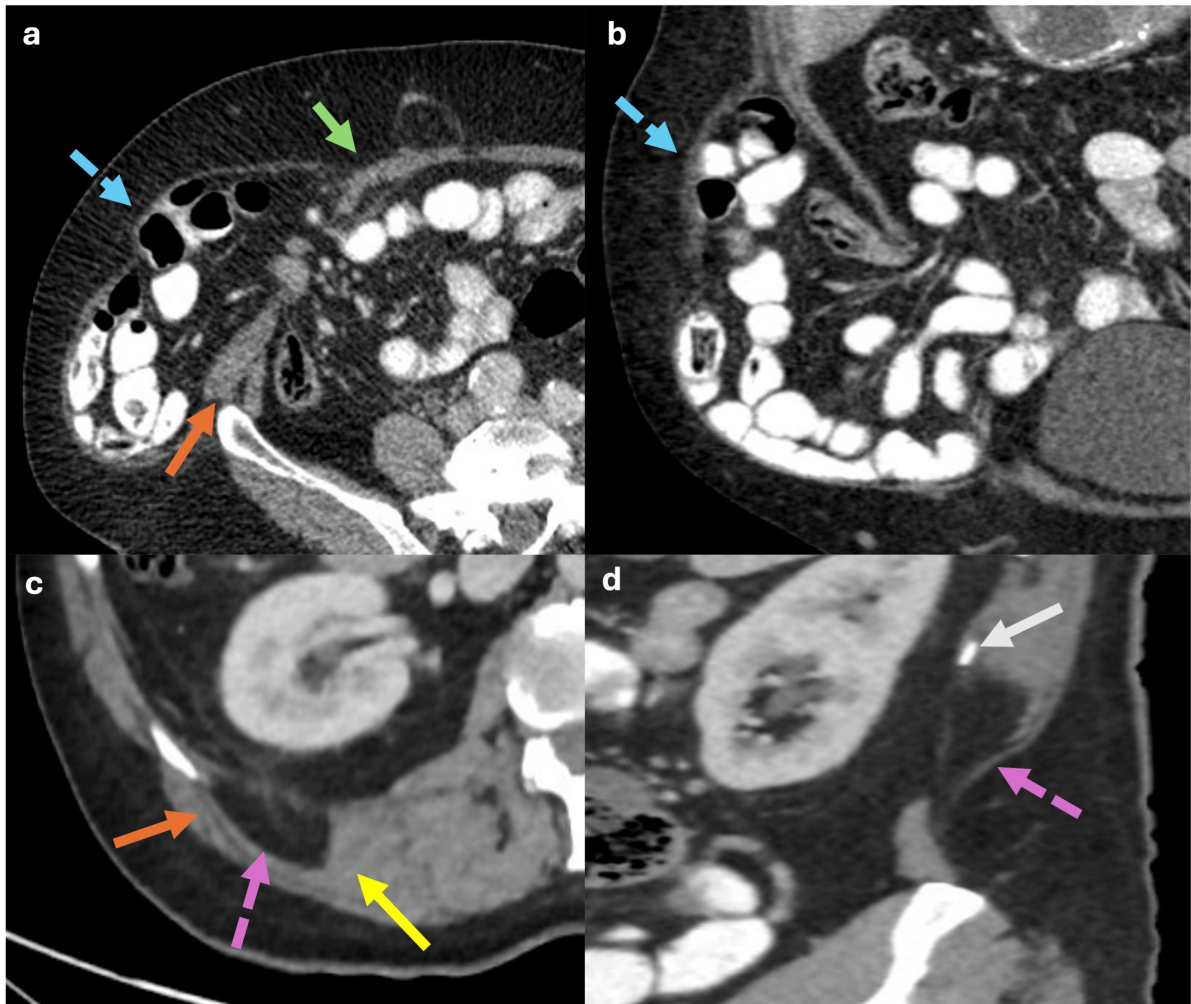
Axial and coronal abdominal CT scan with oral contrast showing a Spigelian hernia and Grynfelt hernia (a, b) Axial and coronal CT scan showing a hernia sac with small bowel loop content (blue dotted arrows) protruding between the rectus abdominis muscle (green arrow) and the internal oblique muscle (orange arrow), consistent with a Spigelian hernia. (c, d) Axial and coronal CT scan from another patient showing a hernia sac with fat content (purple dotted arrows) delimited laterally by the internal oblique muscle (orange arrow), medially by the quadratus lumborum muscle (yellow arrow), and the superiorly by the 12th rib (white arrow) consistent with a Grynfelt hernia in the upper lumbar triangle. The figure was created by the authors.
